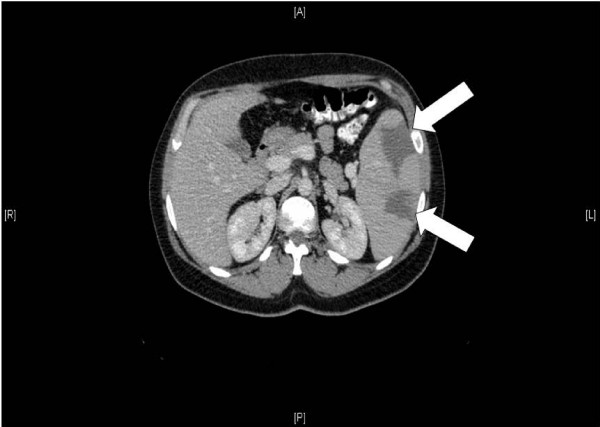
Two large splenic infarcts, as demonstrated on an abdominal CT scan (white arrows).
